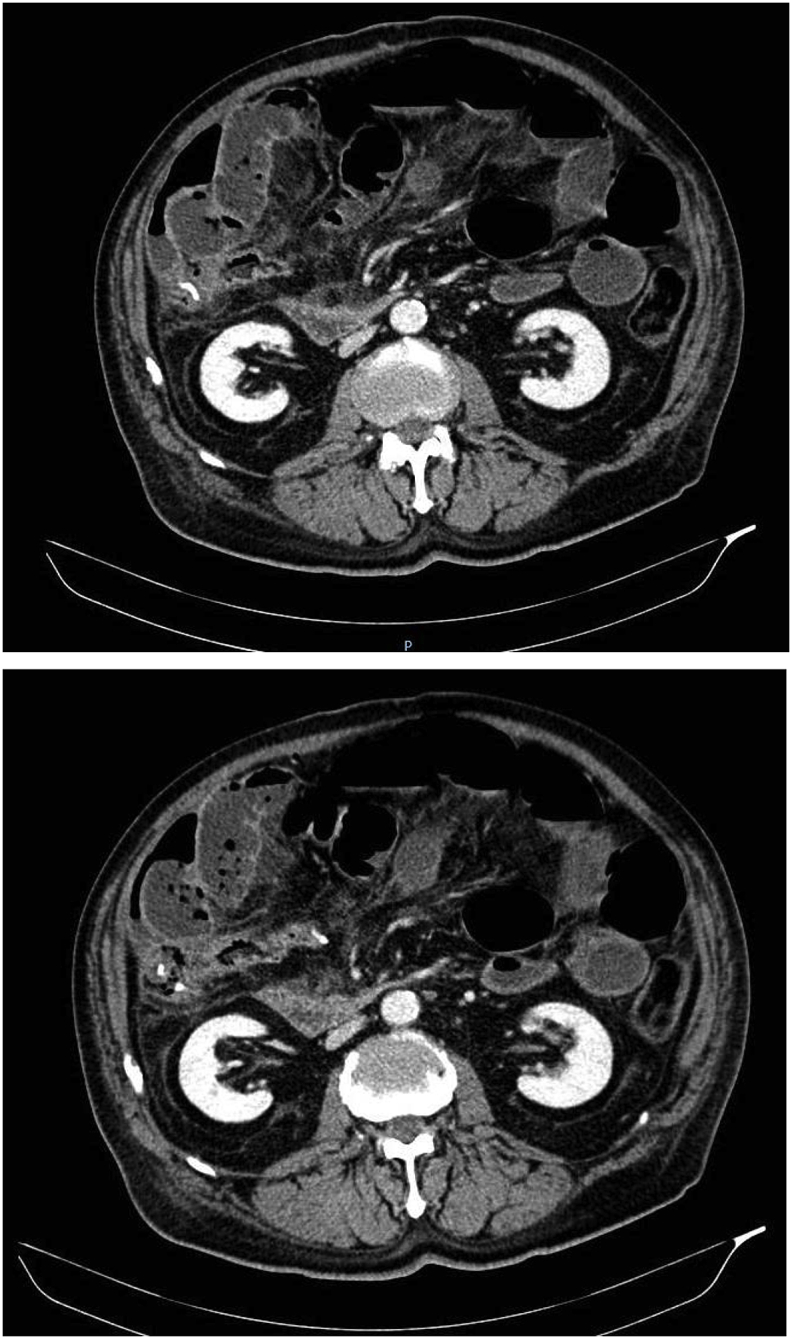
CT scans of the abdomen and pelvis, demonstrating the anstomostic stenosis.
